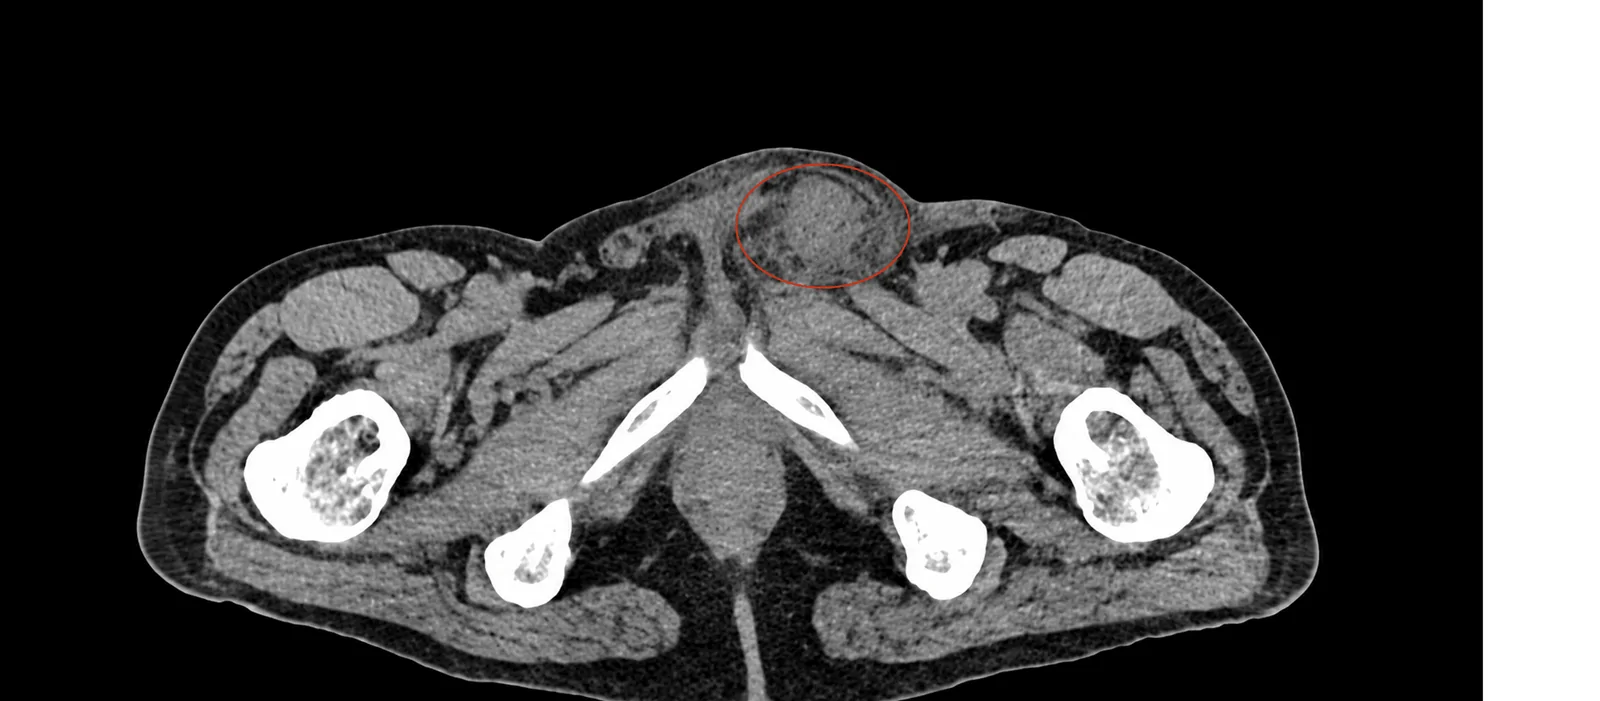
Axial non-contrast computed tomography of the left inguinal region Axial non-contrast CT image demonstrating a 27 × 28 × 53 mm fat-containing soft-tissue lesion within the left inguinal canal (red circle), initially interpreted as an incarcerated recurrent inguinal hernia. The circled lesion shows surrounding inflammatory fat stranding, contributing to the preoperative radiological diagnosis. Surgical exploration subsequently revealed that the lesion corresponded to an abscess adjacent to the vas deferens rather than a recurrent inguinal hernia.
